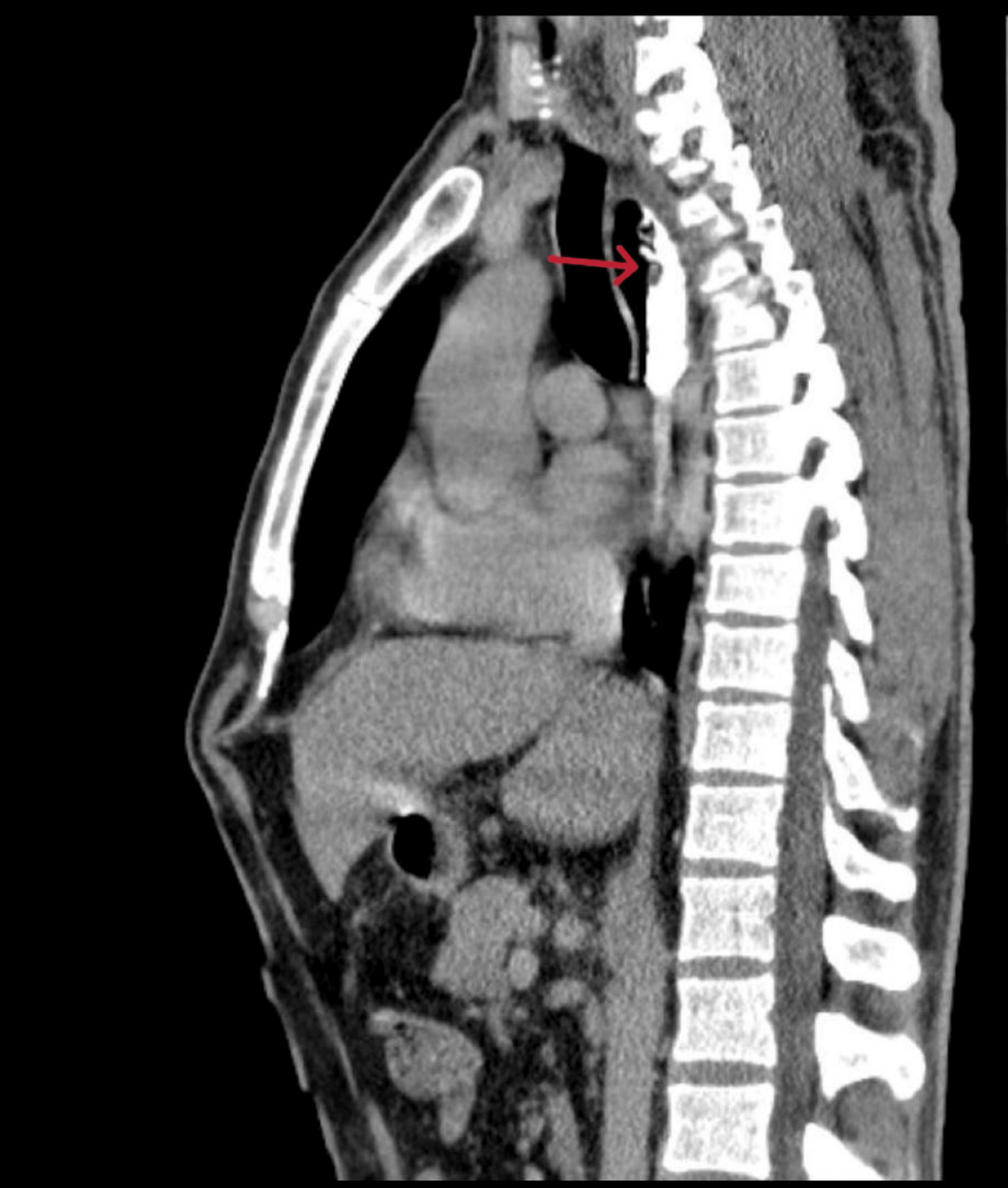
CT thorax showing contrast-filled upper esophagus with mucosal irregularity
